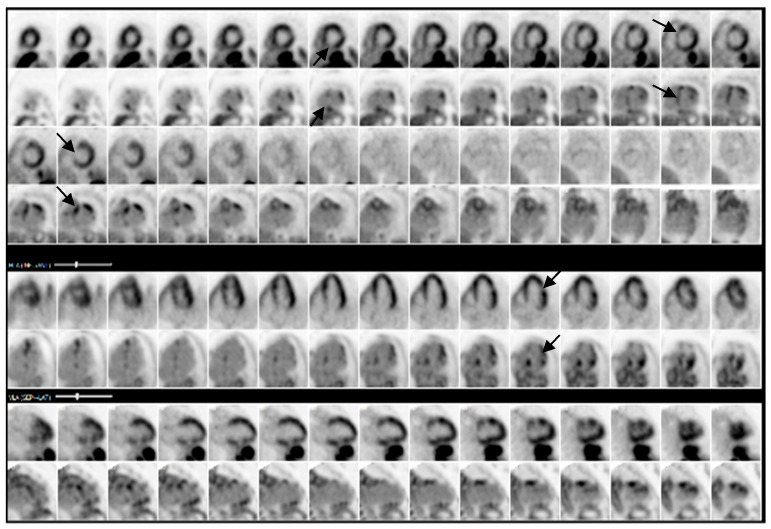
PET demonstrating multi-focal FDG uptake (arrows) with associated matched perfusion abnormalities in the basal and mid-inferior and inferoseptal segments, basal anterior, basal to mid-anterolateral segments, and apical lateral and inferior segments.
